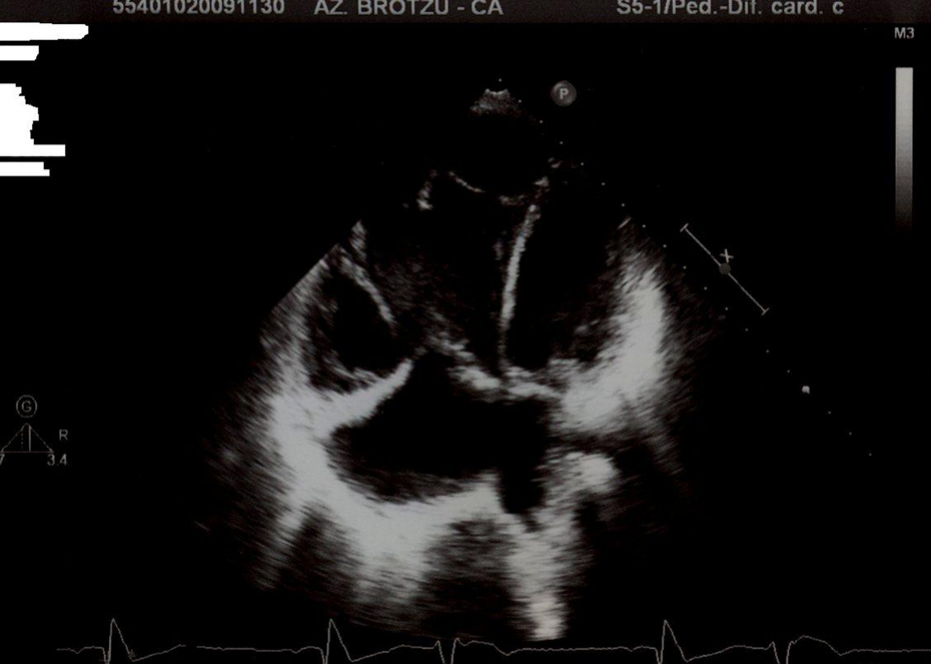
Echocardiographic apical four-chamber view after surgery. Normalization of both the right ventricle and tricuspid valve size. A wide atrial septal defect type ostium secundum is also evident.
